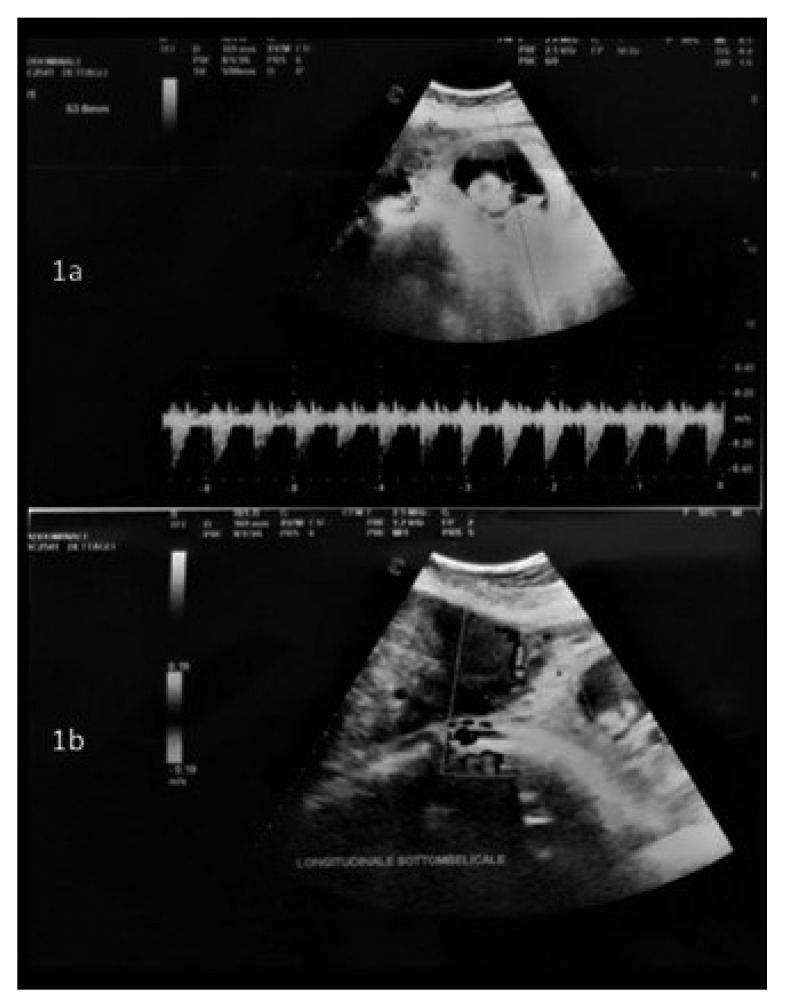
(a) Doppler ultrasound to check fetal heartbeat; (b) Assessment of the position of larger myoma and its pedicle.
